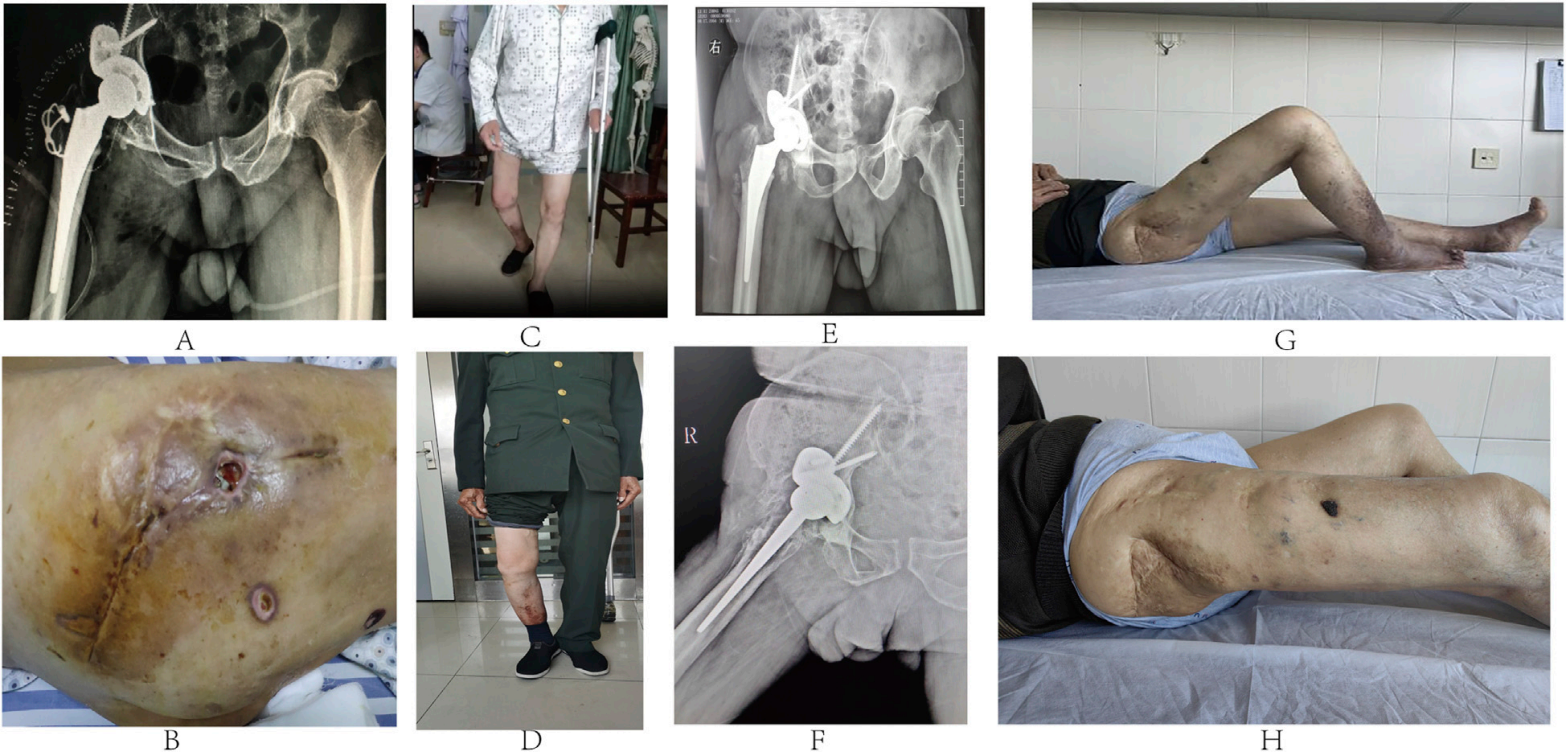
(A) Postoperative pelvic X-rays showing precise prosthesis positioning. (B) Ten days postoperatively, purulent discharge was noted from the incision. (C) Three months postoperatively, he walked with single crutch support. (D) He walked normally 5.5 years postoperatively. (E, F) At 5.5-year follow-up, pelvic X-rays in standard and lateral positions showing artificial joint stability without abnormalities. (G, H) Hip joint range of motion was 0–50° 5.5 years postoperatively.
